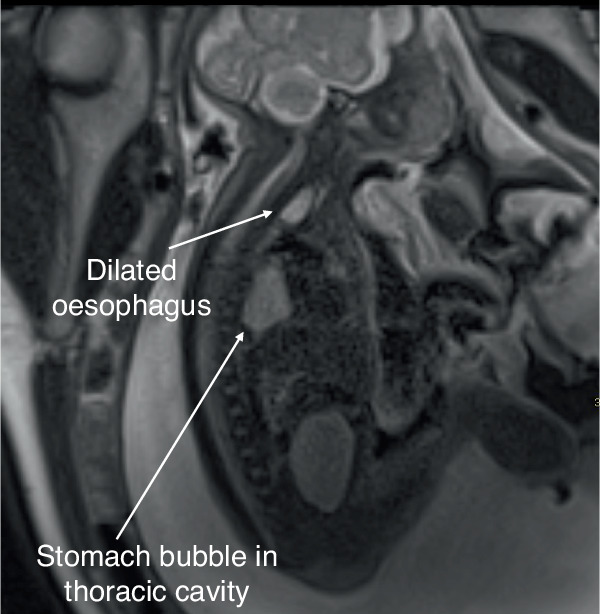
Comorbid TOF/OA and congenital diaphragmatic hernia (CDH). Sagittal T2 SSFSE fetal MRI image of a 35-week fetus with CDH and TOF/OA with rotated stomach bubble in the thoracic cavity and oesophageal pouch.
